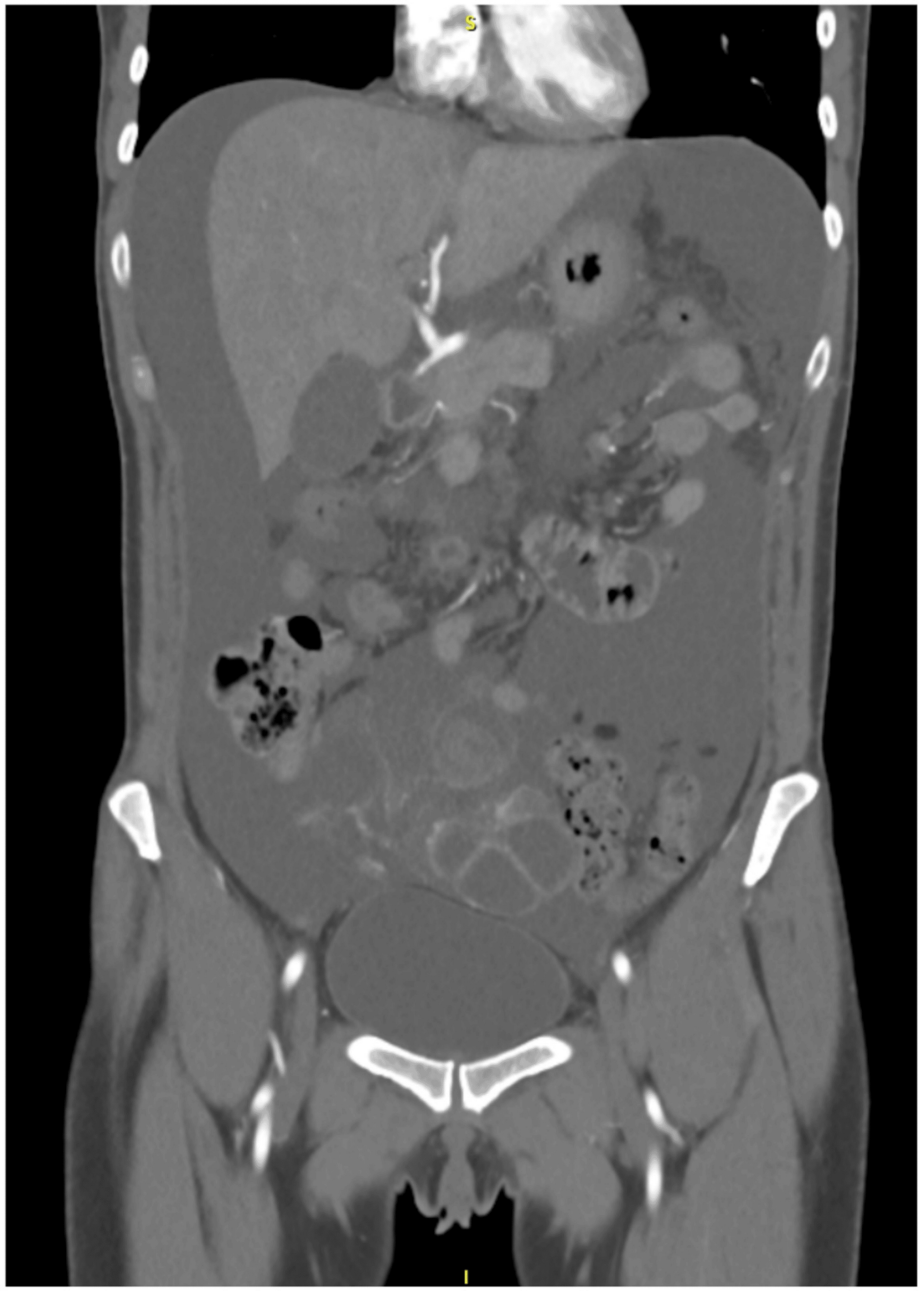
CT of the abdomen and pelvis with IV contrast Showing a large volume of ascites, small pleural effusions, and enlarged bilateral ovaries with multiple theca lutein cysts consistent with ovarian hyperstimulation syndrome.
